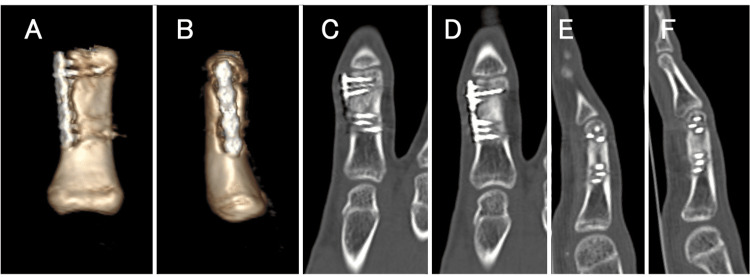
CT images at the three-month follow-up. (A, B, C, D, E, F) CT images at the three-month follow-up showed the ideal position of the proximal phalanx.
